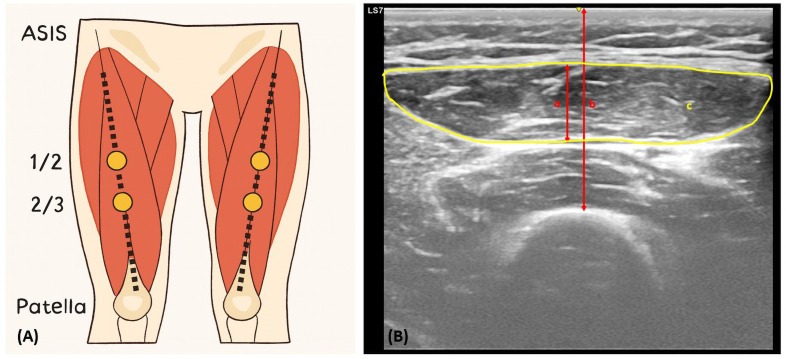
(A) Anatomical landmarks for ultrasound measurements of the rectus femoris at the midpoint (1/2) and distal third (2/3) between the anterior superior iliac spine (ASIS) and the patella. (B) A representative transverse ultrasound image showing the rectus femoris muscle thickness (a), the total anterior thigh muscle thickness (b), and the rectus femoris cross-sectional area (c, yellow contour).
